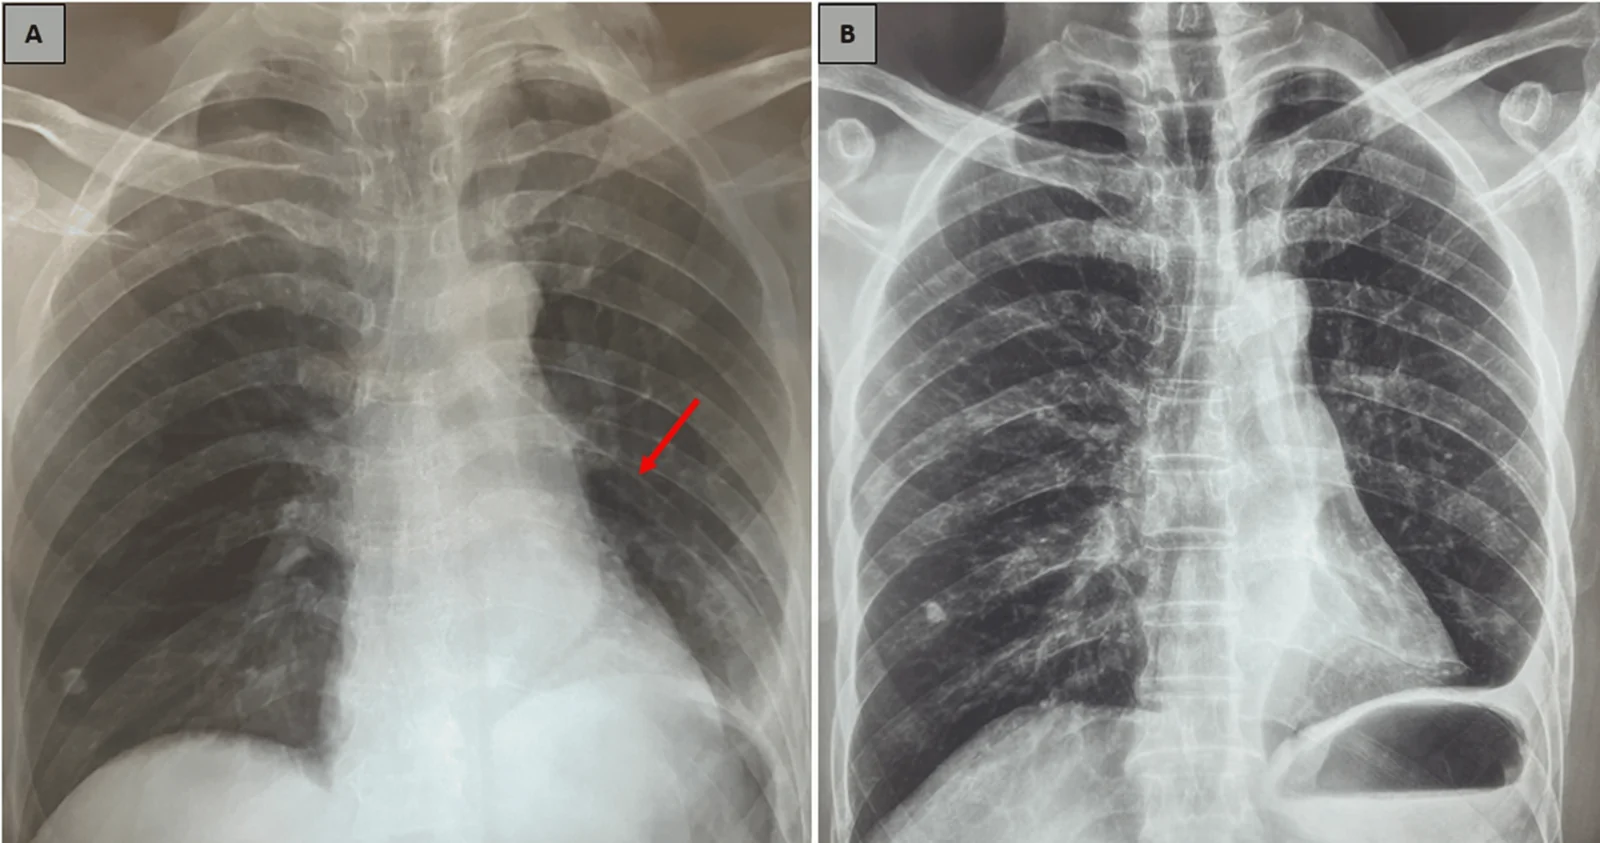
Chest X-ray. (A) Revealed re-expansion of the lung adhered to the wall, with the drain in place (red arrow). (B) Chest X-ray one month later.
